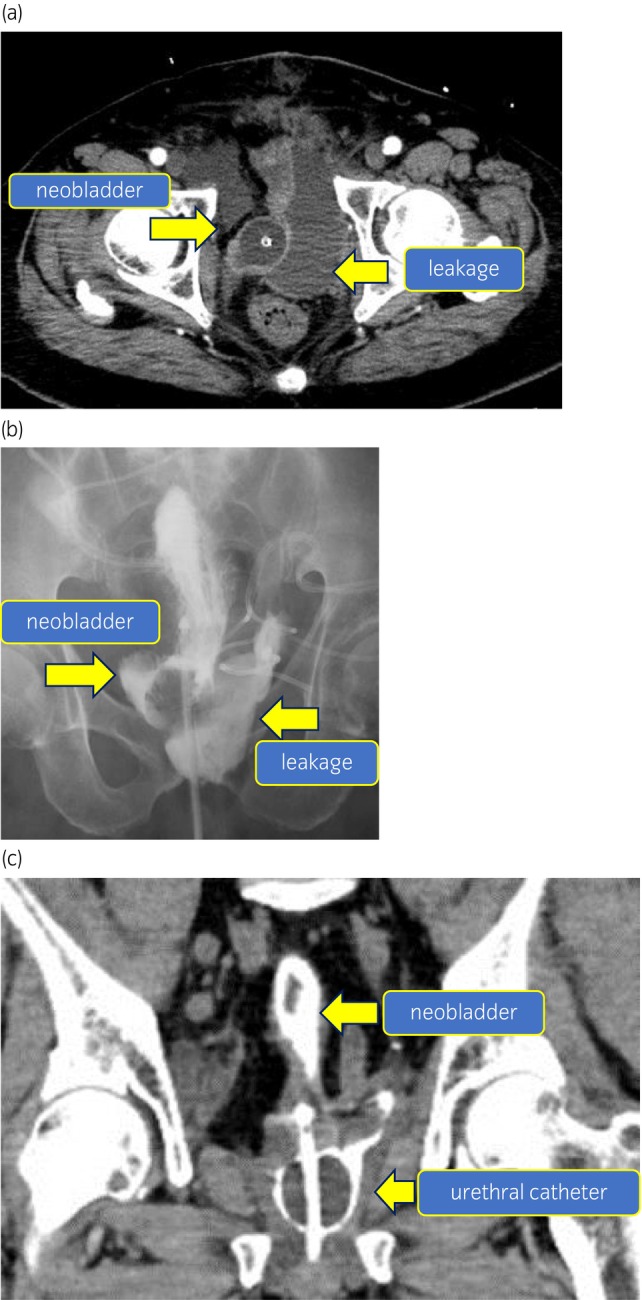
(a) CT image showing the leakage. CT scan of horizontal view for the fever examination revealed large amount of fluid collection on the left side of the urethral‐neobladder anastomosis. This may be a lymphocele infection or leakage of urine. (b) Image of leakage in cystography. Cystography reveals a large amount of leakage on the left side of the anastomosis. It reveals a neobladder‐urothelial anastomotic leakage. (c) CT image of severe leakage. CT scan of the coronary view showing urethral catheter falling off. The urethral balloon is falling off from the neobladder in a CT scan of coronary view. It suggests a severe anastomotic dehiscence of suture.
